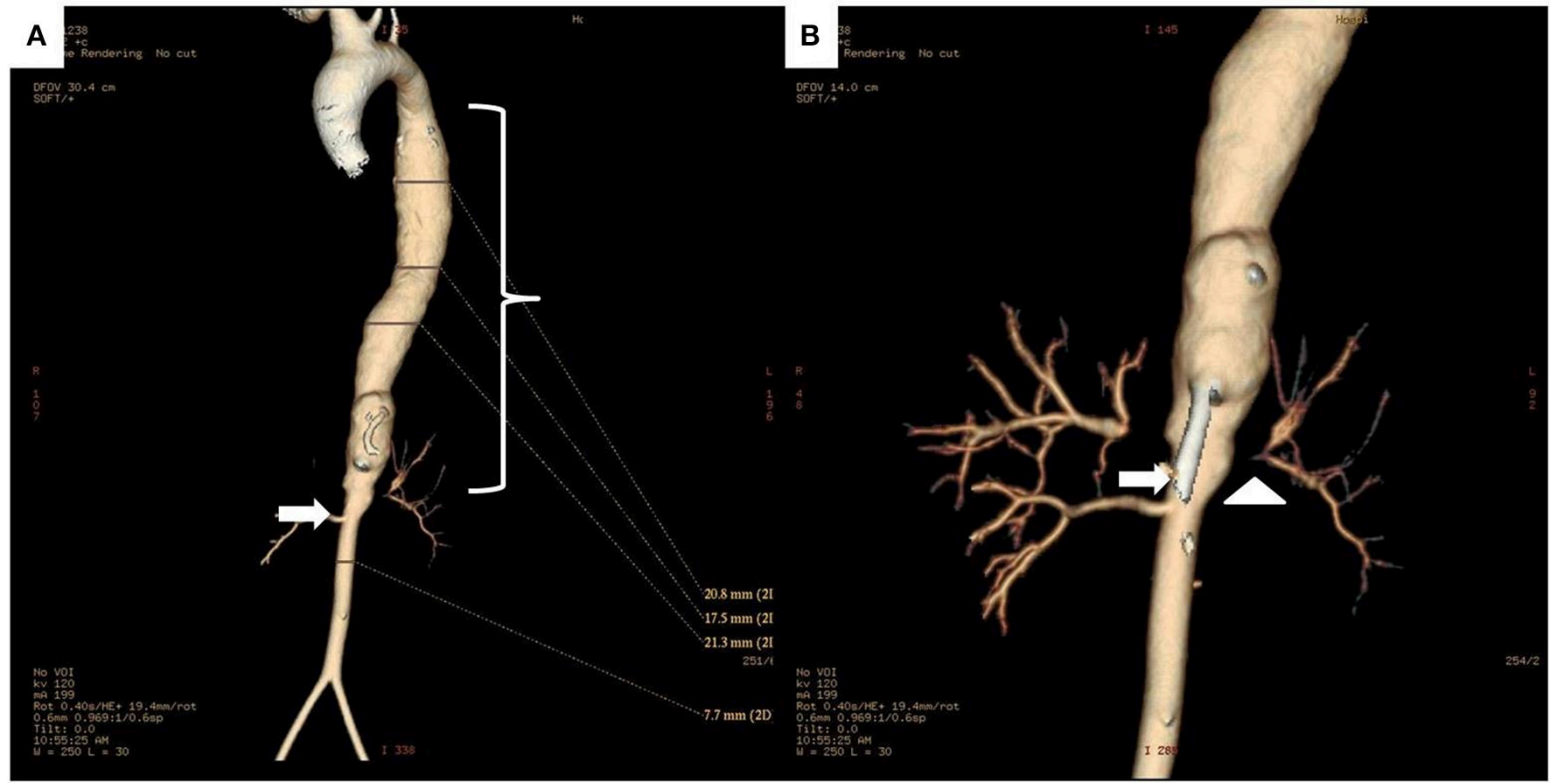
(A) 3D reconstruction CT images of the whole aorta in a 12 year-old female Takayasu arteritis patient with thoraco-abdominal aortic aneurysm (bracket) proximal to a stenotic lesion (arrow) at the renal artery emergence site. (B) Detail of the aneurysmal dilatation and stenosis of the abdominal aorta (arrow), and stenosis of left renal artery (arrowhead).
